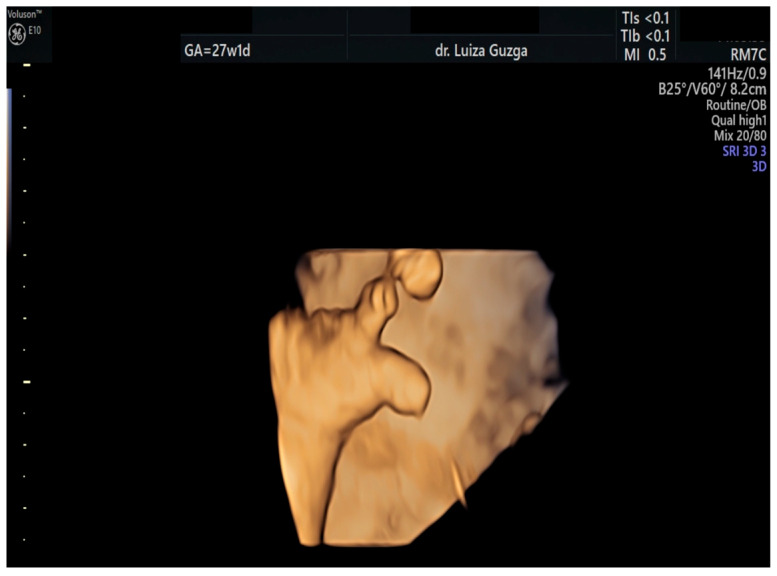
Three-dimensional ultrasound assessment of the fetal hand at 27 weeks of gestation, revealing a short thumb. Abbreviations: GA (gestational age); w (weeks); d (days).
